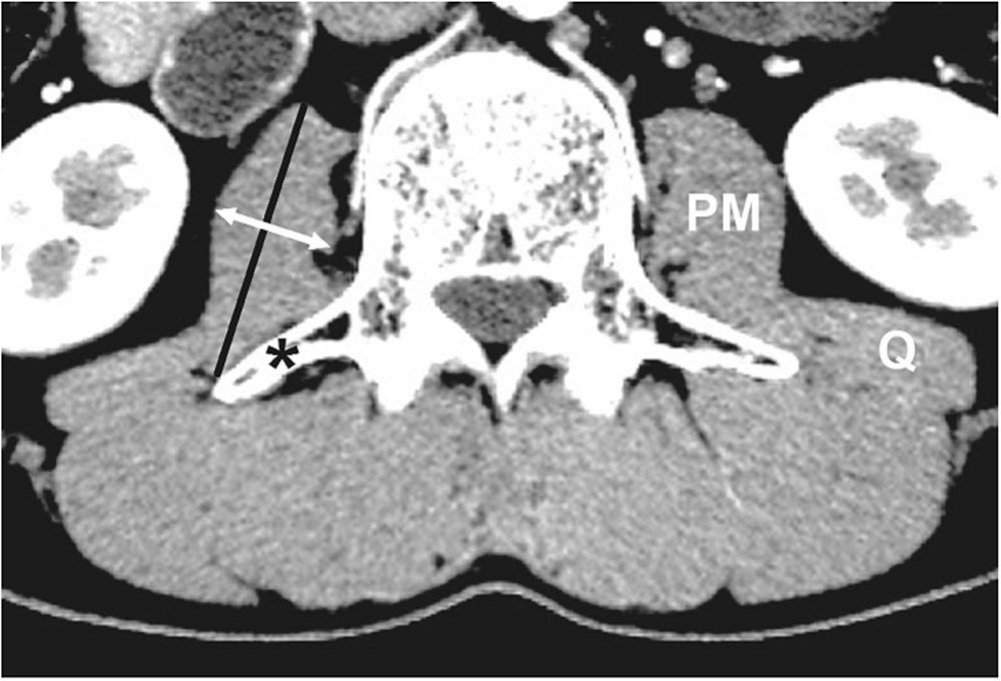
Psoas muscle measurement with a cross-sectional CT image. White arrow, psoas muscle thickness (PMT); black line, antero-posterior axis of the psoas muscle; asterisk, the transverse process of the 3rd lumber vertebra; Q, quadratus lumborum muscle.
